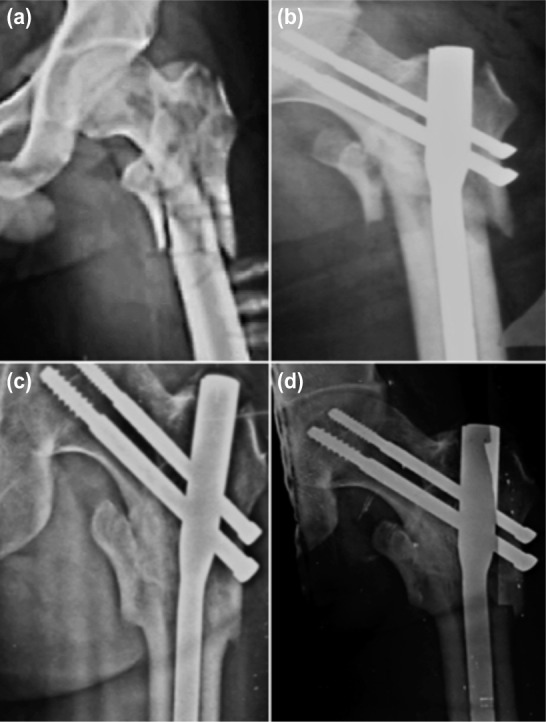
AP radiographs (a) Pre-op, (b) Post-op, (c) three month follow-up and (d) six month follow-up.
